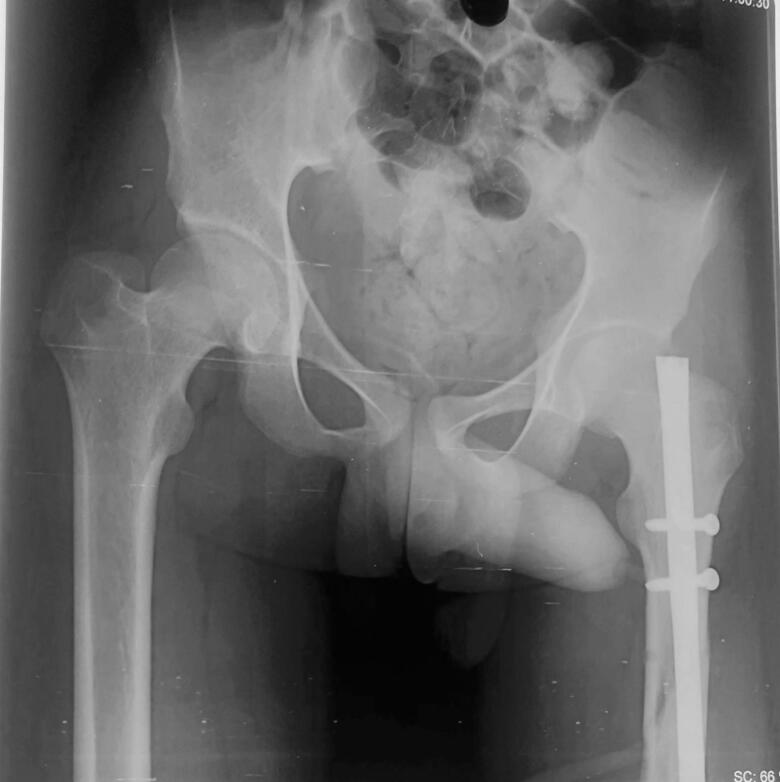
Post-operative day 14 x-ray pelvis with bilateral hip satisfactory.
